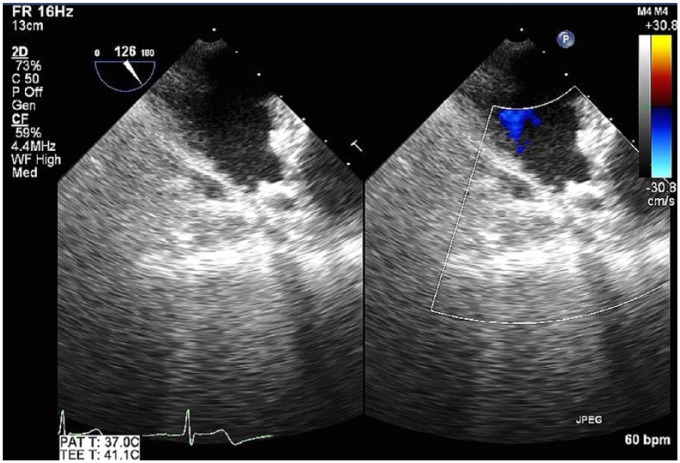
Transesophageal echocardiography images with color Doppler 2 days after device placement shows Watchman deep in the left atrial appendage, without significant peri-device leak. Fluid is no longer apparent in the transverse sinus.
